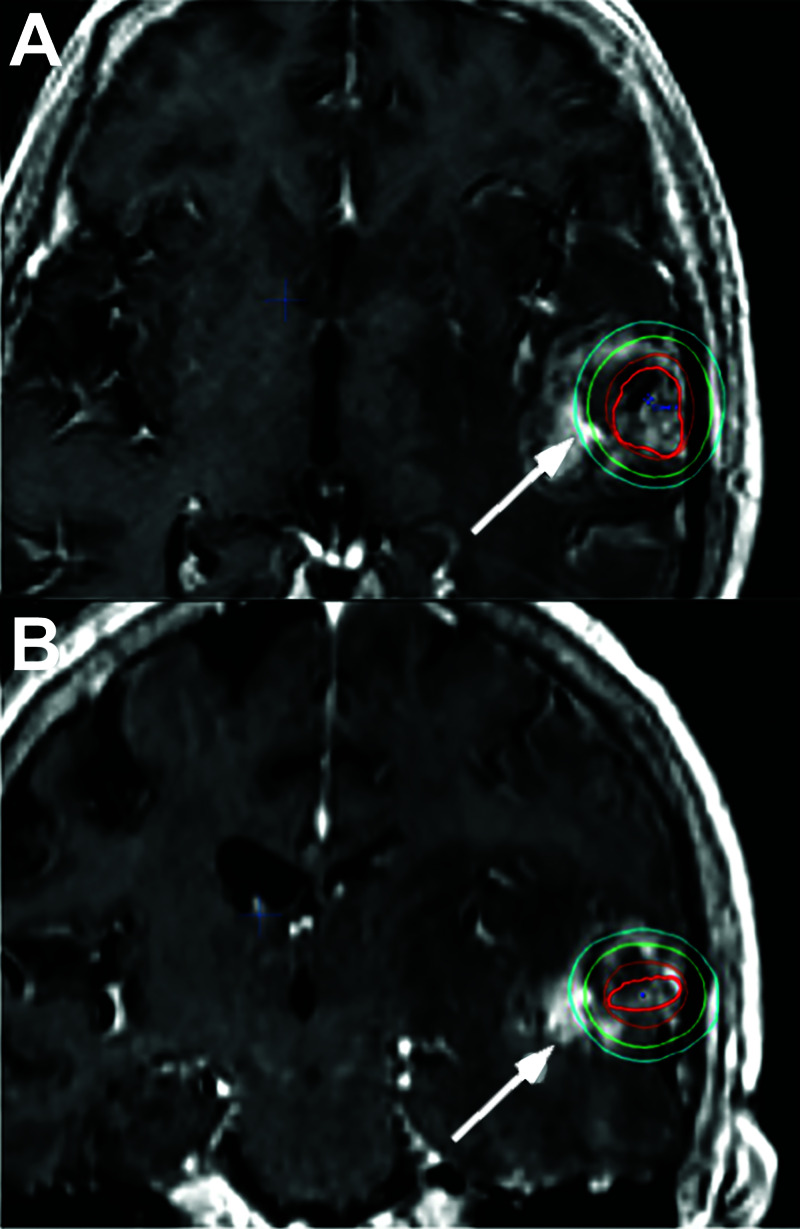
T1-weighted post-contrast magnetic resonance imaging axial (A) and coronal (B) scans from a patient before stereotactic radiosurgery (SRS) for arteriovenous malformation, superimposed with the radiotherapy doses (arrows) The left temporal lesion was targeted with a prescription dose of 20 Gy. The red ovoid line demonstrates the target of SRS. Isodense lines surrounding the target lesion represent a gradient of dose dissipation: 20 Gy (brown), 12 Gy (emerald), and 8 Gy (turquoise).
